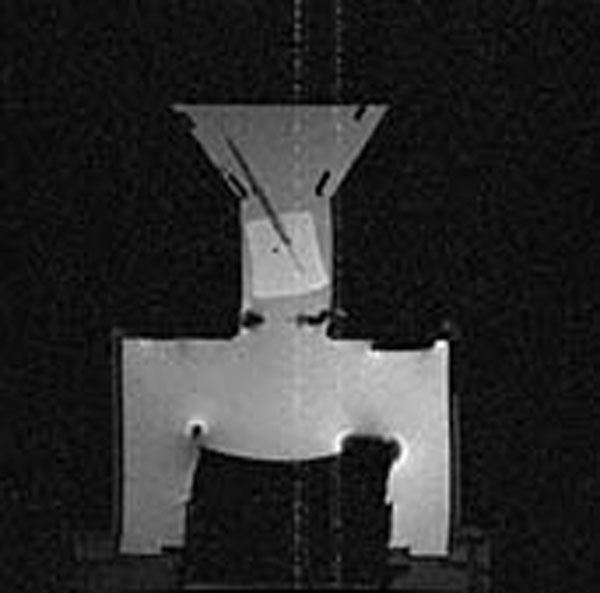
MRI Image of the chamber with the tissue showing the thermocouple tip at the ultrasound focus
